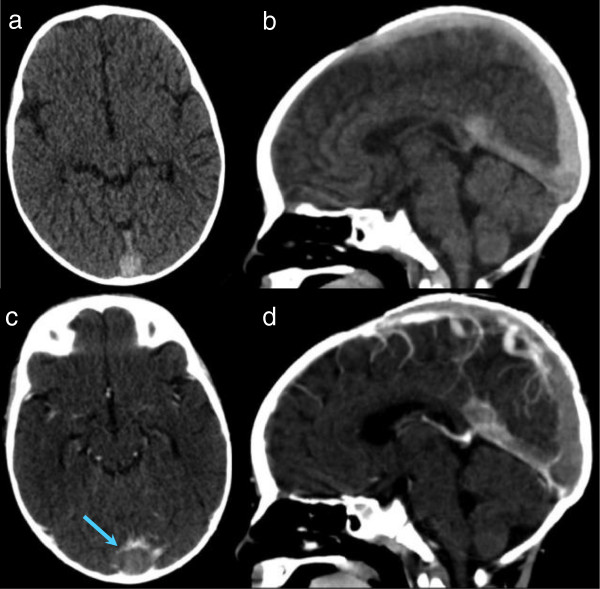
Head computed tomography images without (a,b) and with (c,d) contrast; axial (a,c) and reformatted sagittal (b,d) images. Note the empty delta sign (c, arrow).
